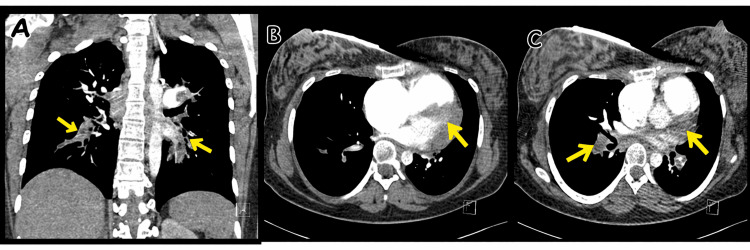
Computed tomography pulmonary angiography demonstrating extensive bilateral pulmonary embolism with central and segmental filling defects (arrows), consistent with a high clot burden.
